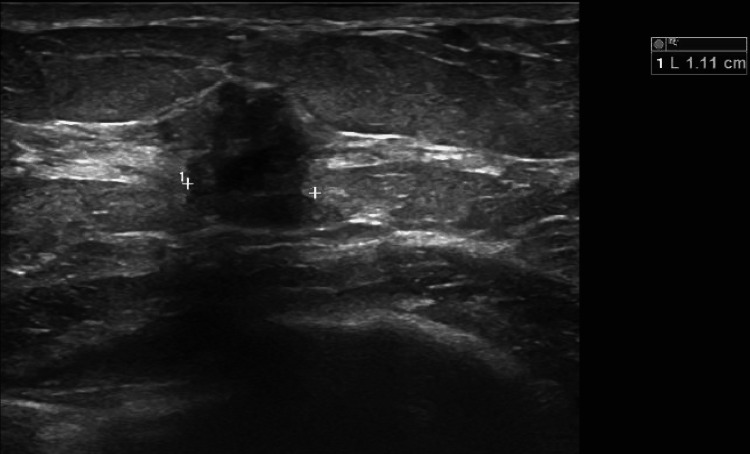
Ultrasound of the six o'clock mass Ultrasound findings stated that "The 1.5 cm x 1.2 cm x 1.1 cm mass in the right breast at six o'clock anterior depth appears suspicious of malignancy."
